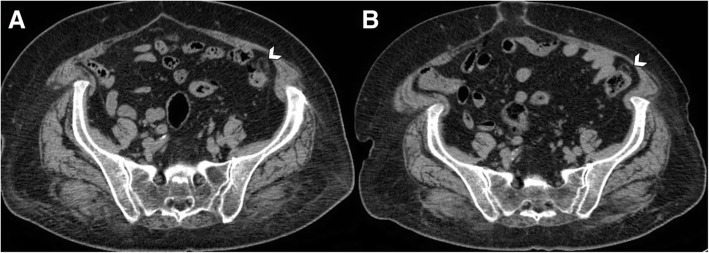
Evolution of acute epiploic appendagitis in a 72-year-old woman. a Axial non-contrast CT image shows a pericolonic fat-density lesion (arrowhead) that abuts anteriorly the colon-sigmoid junction in the left iliac fossa. b At 3-month follow-up, CT image demonstrates reduction in size of the lesion (arrowhead)
